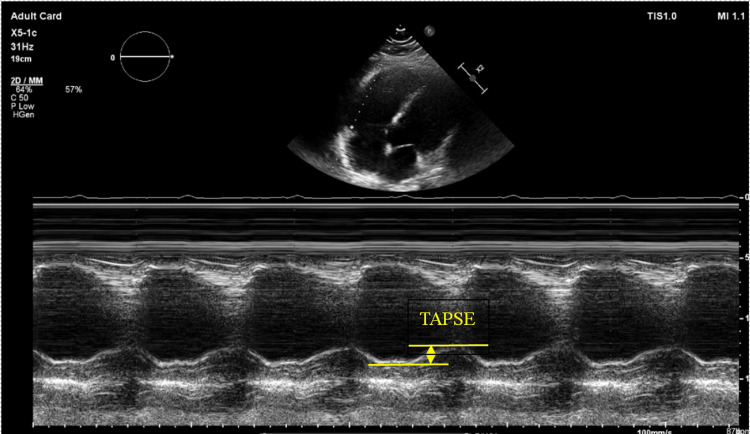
Echocardiogram demonstrating TAPSE. Tricuspid annular plane systolic excursion (TAPSE) is an echocardiographic measure that indicates right ventricular (RV) function. The image above demonstrates a TAPSE of 1.45 cm (normal >1.7 cm), indicating RV dysfunction in this patient.
